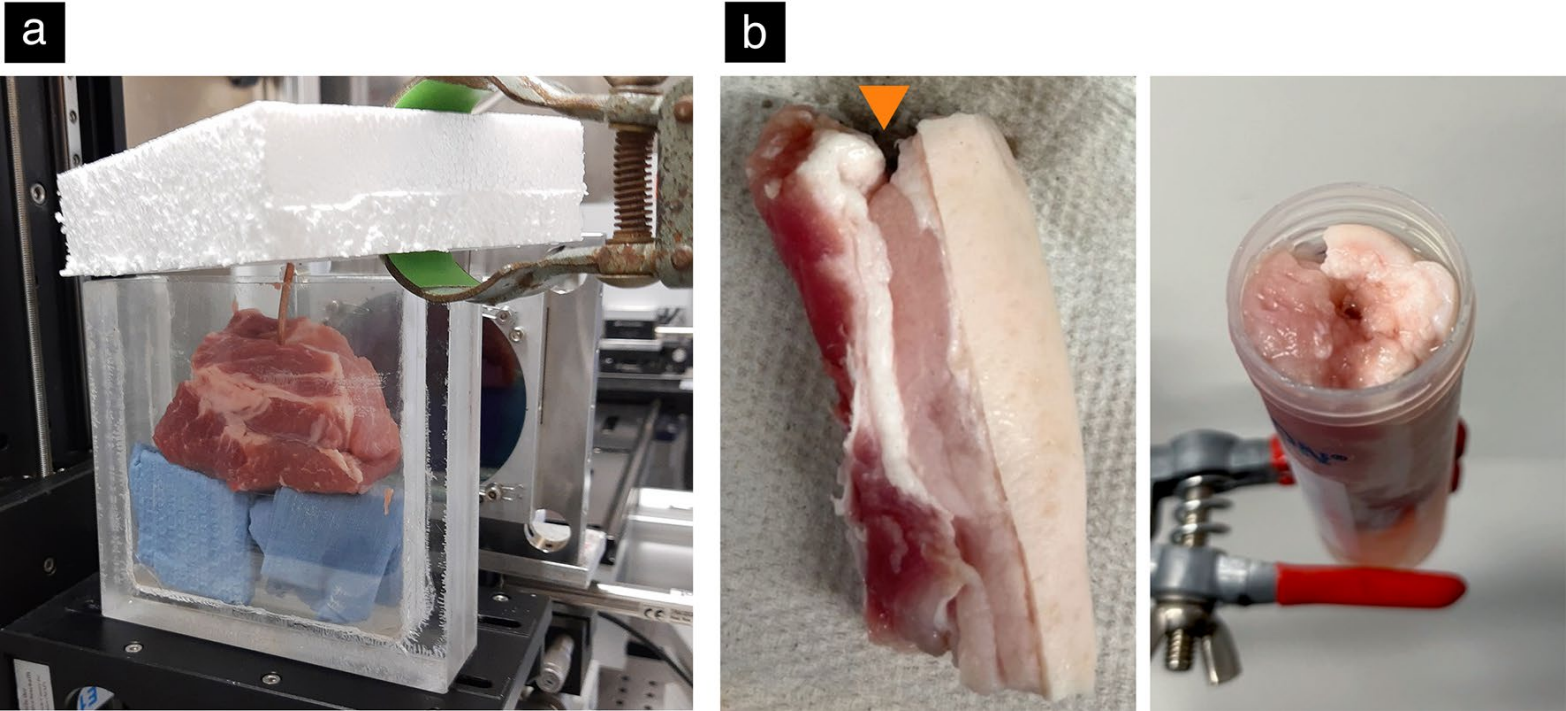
Photographs of the samples used in the experiments. (a) Piece of pork neck in measurement position, as used for the radiography experiment. The inserted copper needle is visible in the middle of the sample; it is cooled using the polystyrene basin on top of the polycarbonate sample holder. (b) Piece of pork belly used for the CT experiment after removal of the cooling tube. The orange arrow indicates the top-facing part of the sample.
